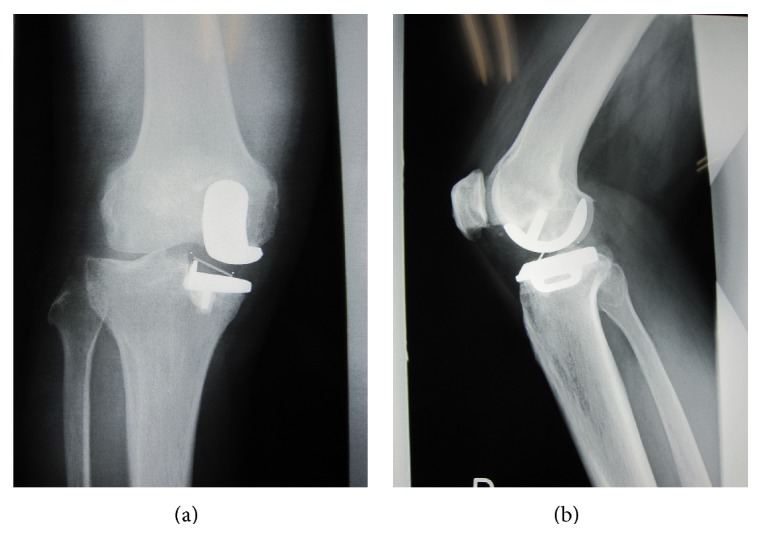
Anteroposterior (a) and lateral (b) weight-bearing radiographs showing the abnormal position of marker in meniscal bearing (case 2).
